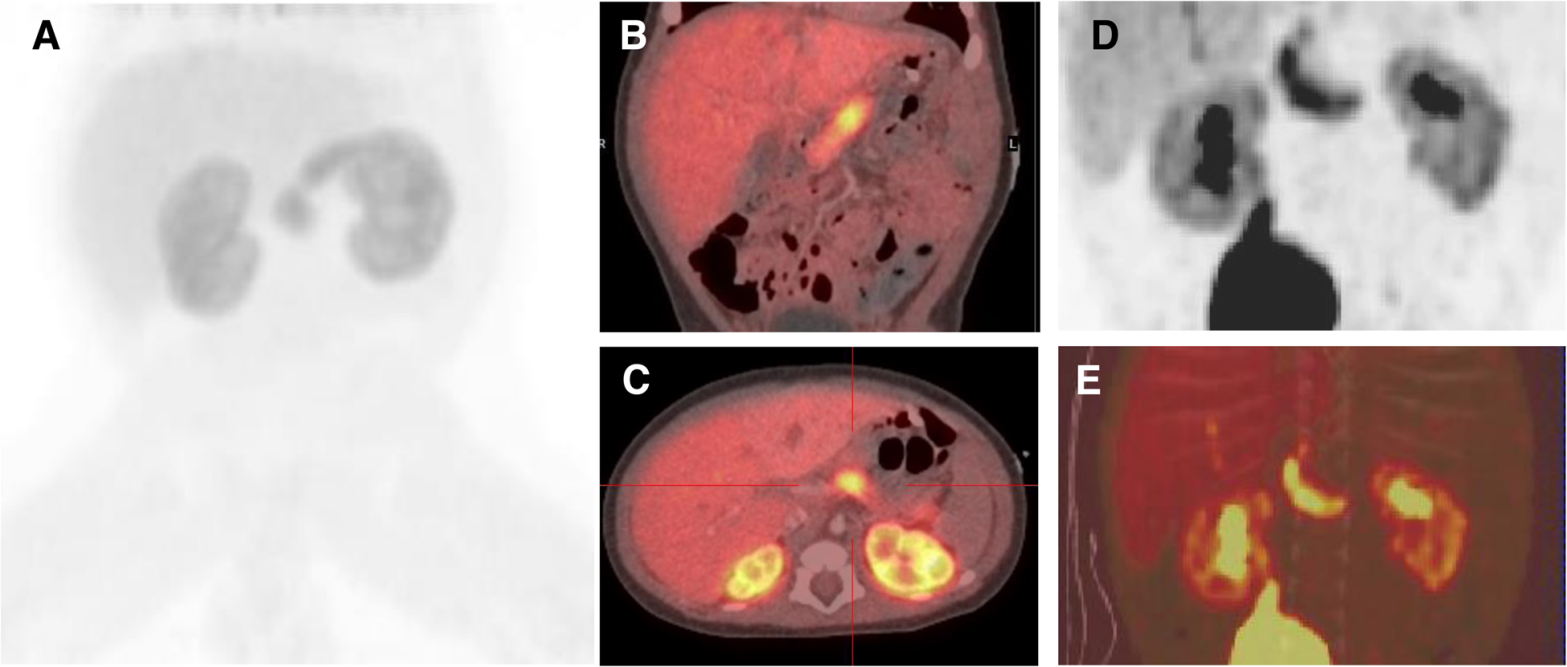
^18^F-DOPA PET/CT Pancreatic Imaging. ^18^F-DOPA PET/CT imaging in the case described demonstrates diffuse pancreatic uptake (a-c). A coronal maximal intensity projection (MIP) image with OSEM reconstruction is shown at 30–45 min after radionucleotide injection (a) with merged coronal (b) and axial (c) PET and CT images. The previous patient with the same *KCNJ11* mutation [7] demonstrated similar findings on ^18^F-DOPA PET-CT scanning with diffuse uptake throughout the pancreas at 44 min post-injection on both MIP and merged PET-CT coronal images (d, e)
